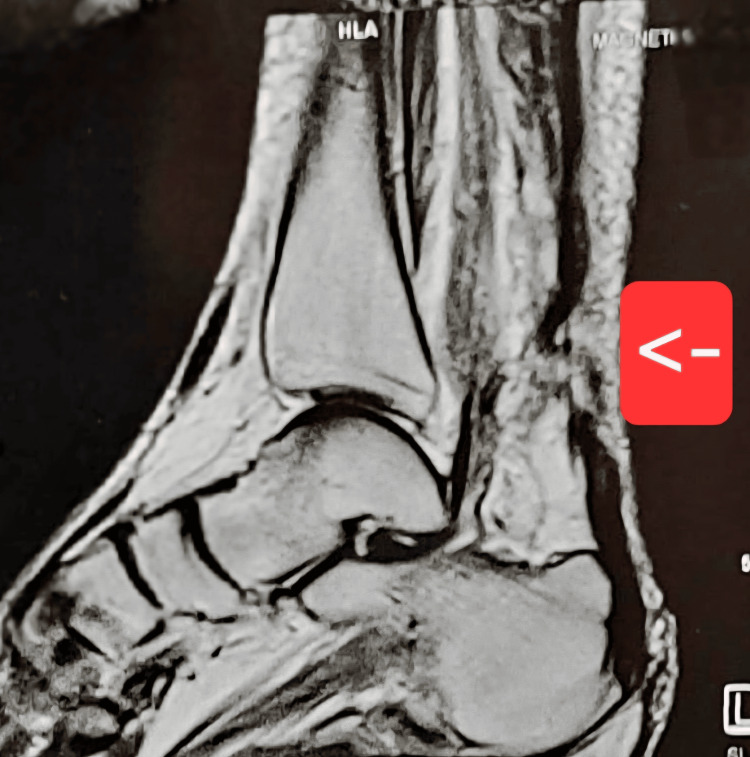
Left Ankle MRI (Sagittal View) Demonstrating Chronic Achilles Tendon Rupture Sagittal MRI of the left ankle demonstrating complete discontinuity of the Achilles tendon (arrow) with proximal tendon retraction and interposed degenerative tissue, consistent with a chronic rupture. The tendon defect (gap size) is estimated to exceed 5 cm.
